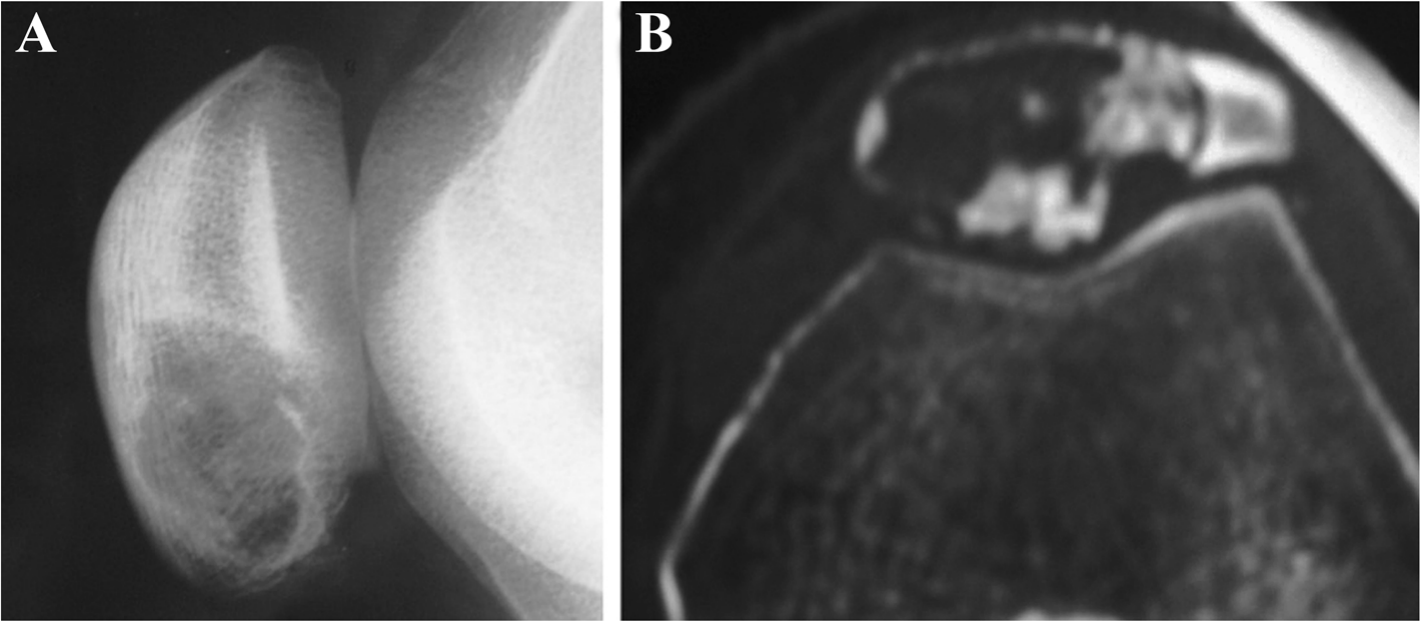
Chondroblastomas of the patella. (A) On plain radiographs, the lesion appears as geographic changes with lobulated margins, thinning cortex, sclerotic rim, and reactive bone. (B) Calcifications inside the lesion are seen on CT images.
